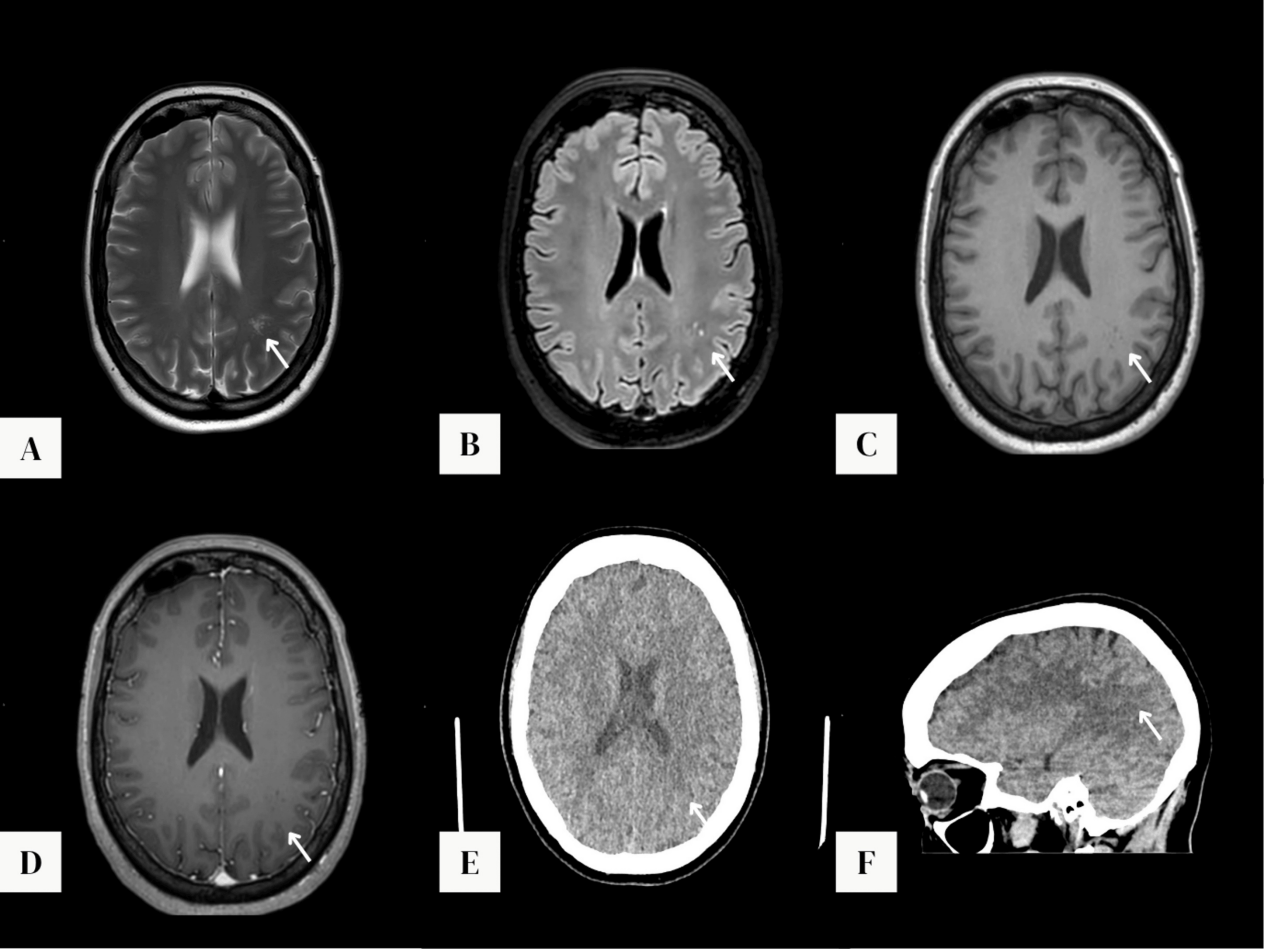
Imaging characteristics of a multinodular and vacuolating neuronal tumor in the left parietal lobe: MRI and CT features. Axial T2 image (A) and axial fluid-attenuated inversion recovery image (B) demonstrate a subcortical cluster of hyperintense nodules in the left parietal lobe (white arrows). Pre-contrast T1 (C) and post-contrast T1 (D) images show a cluster of slightly hypointense lesions in the grey matter without enhancement (white arrows). Brain CT in axial (E) and sagittal (F) planes reveals a hypodense area in the left parietal lobe with subcortical topography, with partially lobulated contour, such as a cluster of hypodense nodules (white arrows).
